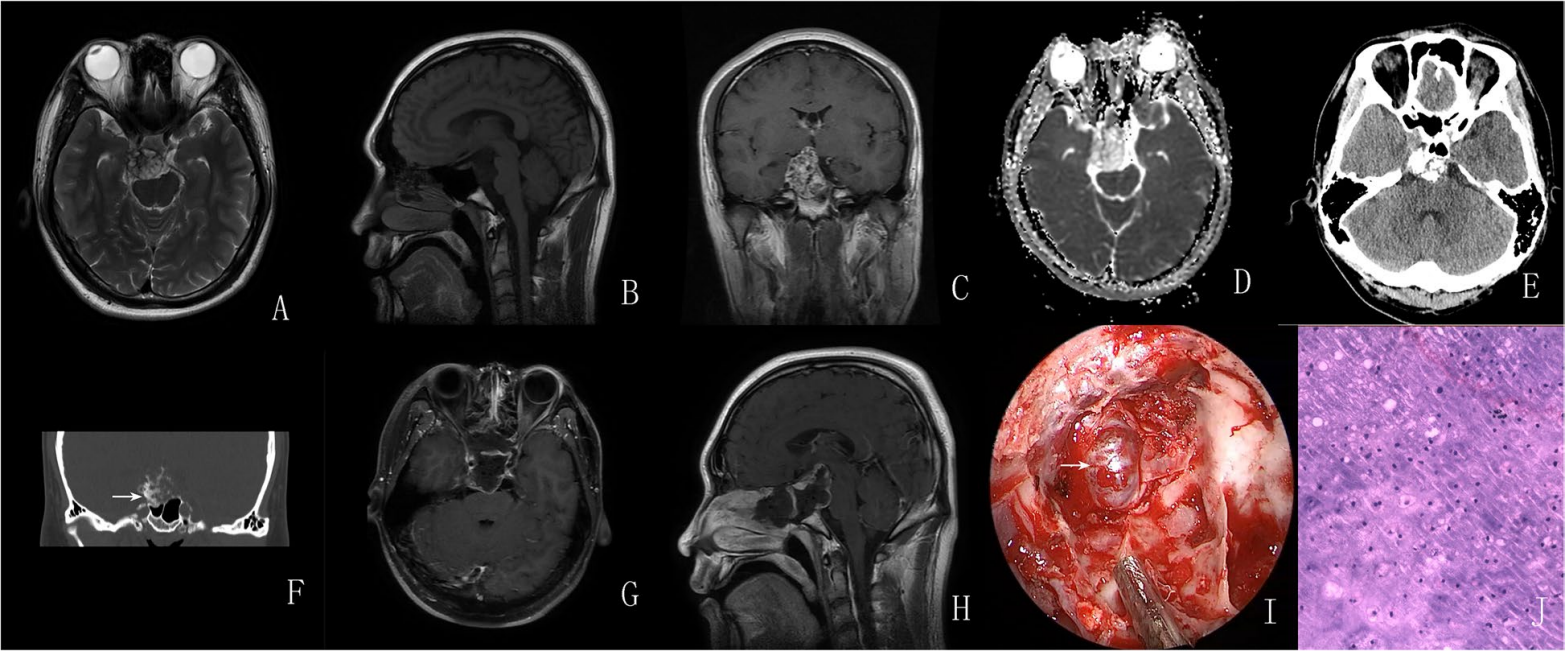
A–C Preoperative MRI revealed an oval lesion in the intrasellar and suprasellar region with mixed signal intensity on T2-weighted imaging, hypointensity on T1-weighted imaging, and heterogeneous enhancement following the administration of gadolinium. D ADC sequence showed no diffusion restriction in the tumor. E–F CT scan showed that tumor contained an irregular calcified nodule; the bone window setting showed bony destruction and bony attachment (arrow). G–H Postoperative MRI showed that tumor complete resection was achieved using an endonasal endoscopic approach. I: Intraoperatively, the tumor was extradural with close adhesion to the clivus dura mater and the dura mater was intact (arrow). J Pathological examination confirmed the diagnosis of chondrosarcoma with immunopositivity for Vimentin and S-100
